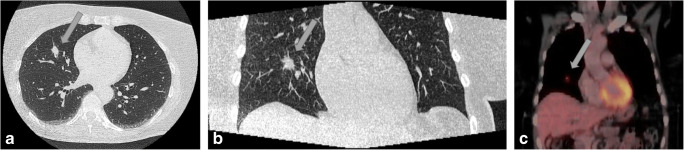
Incidentally detected poorly differentiated acinar adenocarcinoma of the lung in a 61-year-old woman with atypical chest pain. a Spiculated 9 × 14 mm consolidation in segment 5 of the right lung in CTA (*arrow*), axial plane. b CTA, coronal plane. c Subsequent PET-CT examination confirms lung cancer (*arrow*)
